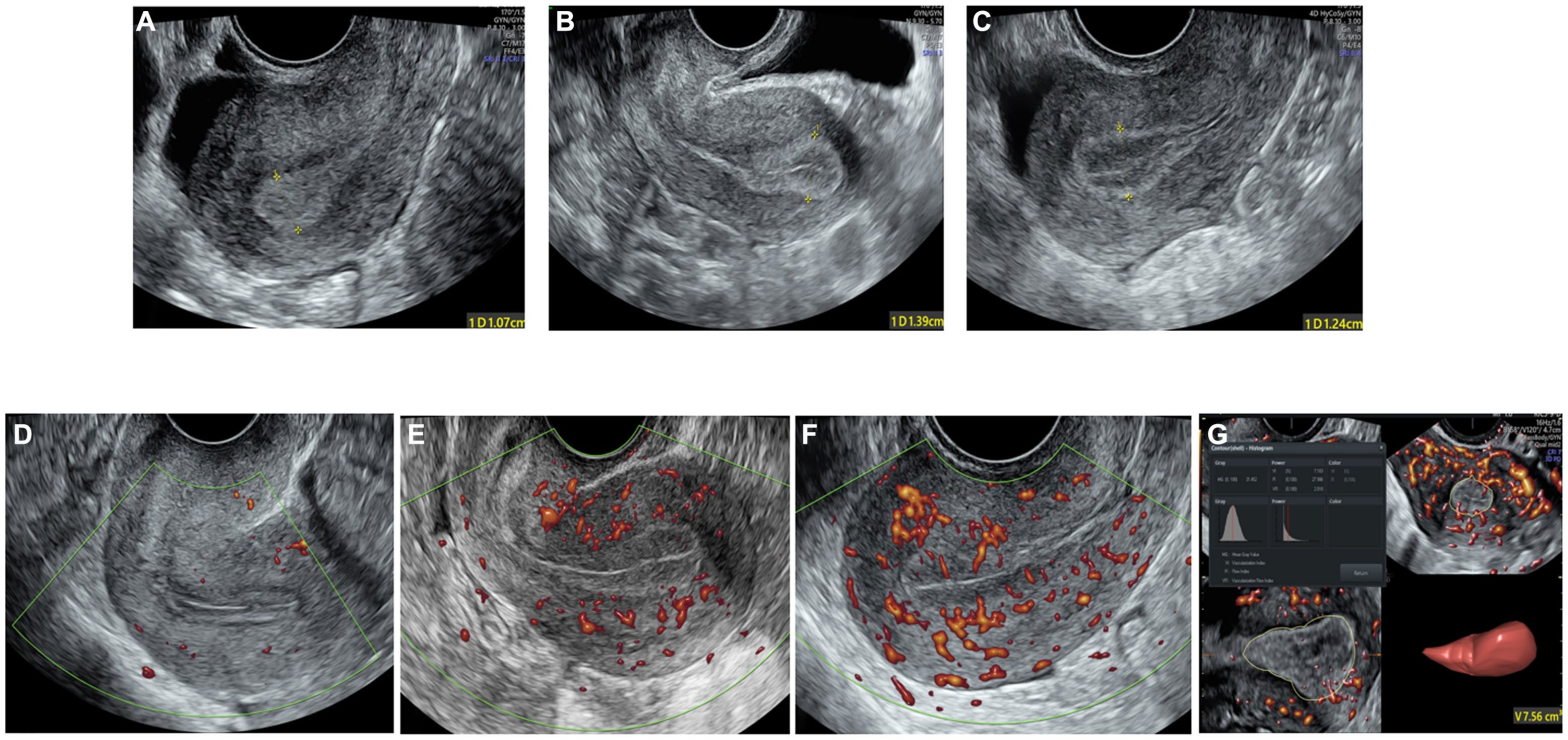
The two-dimensional grayscale image shows the endometrial morphology. (A) Type A endometrial pattern; (B) type B endometrial pattern; (C) type C endometrial pattern. Power Doppler shows the blood flow pattern of the endometrium. (D) Blood flow pattern I; (E) blood flow pattern II; (F) blood flow pattern III. (G) Three-dimensional image showing the vascularization parameters and endometrial volume.
